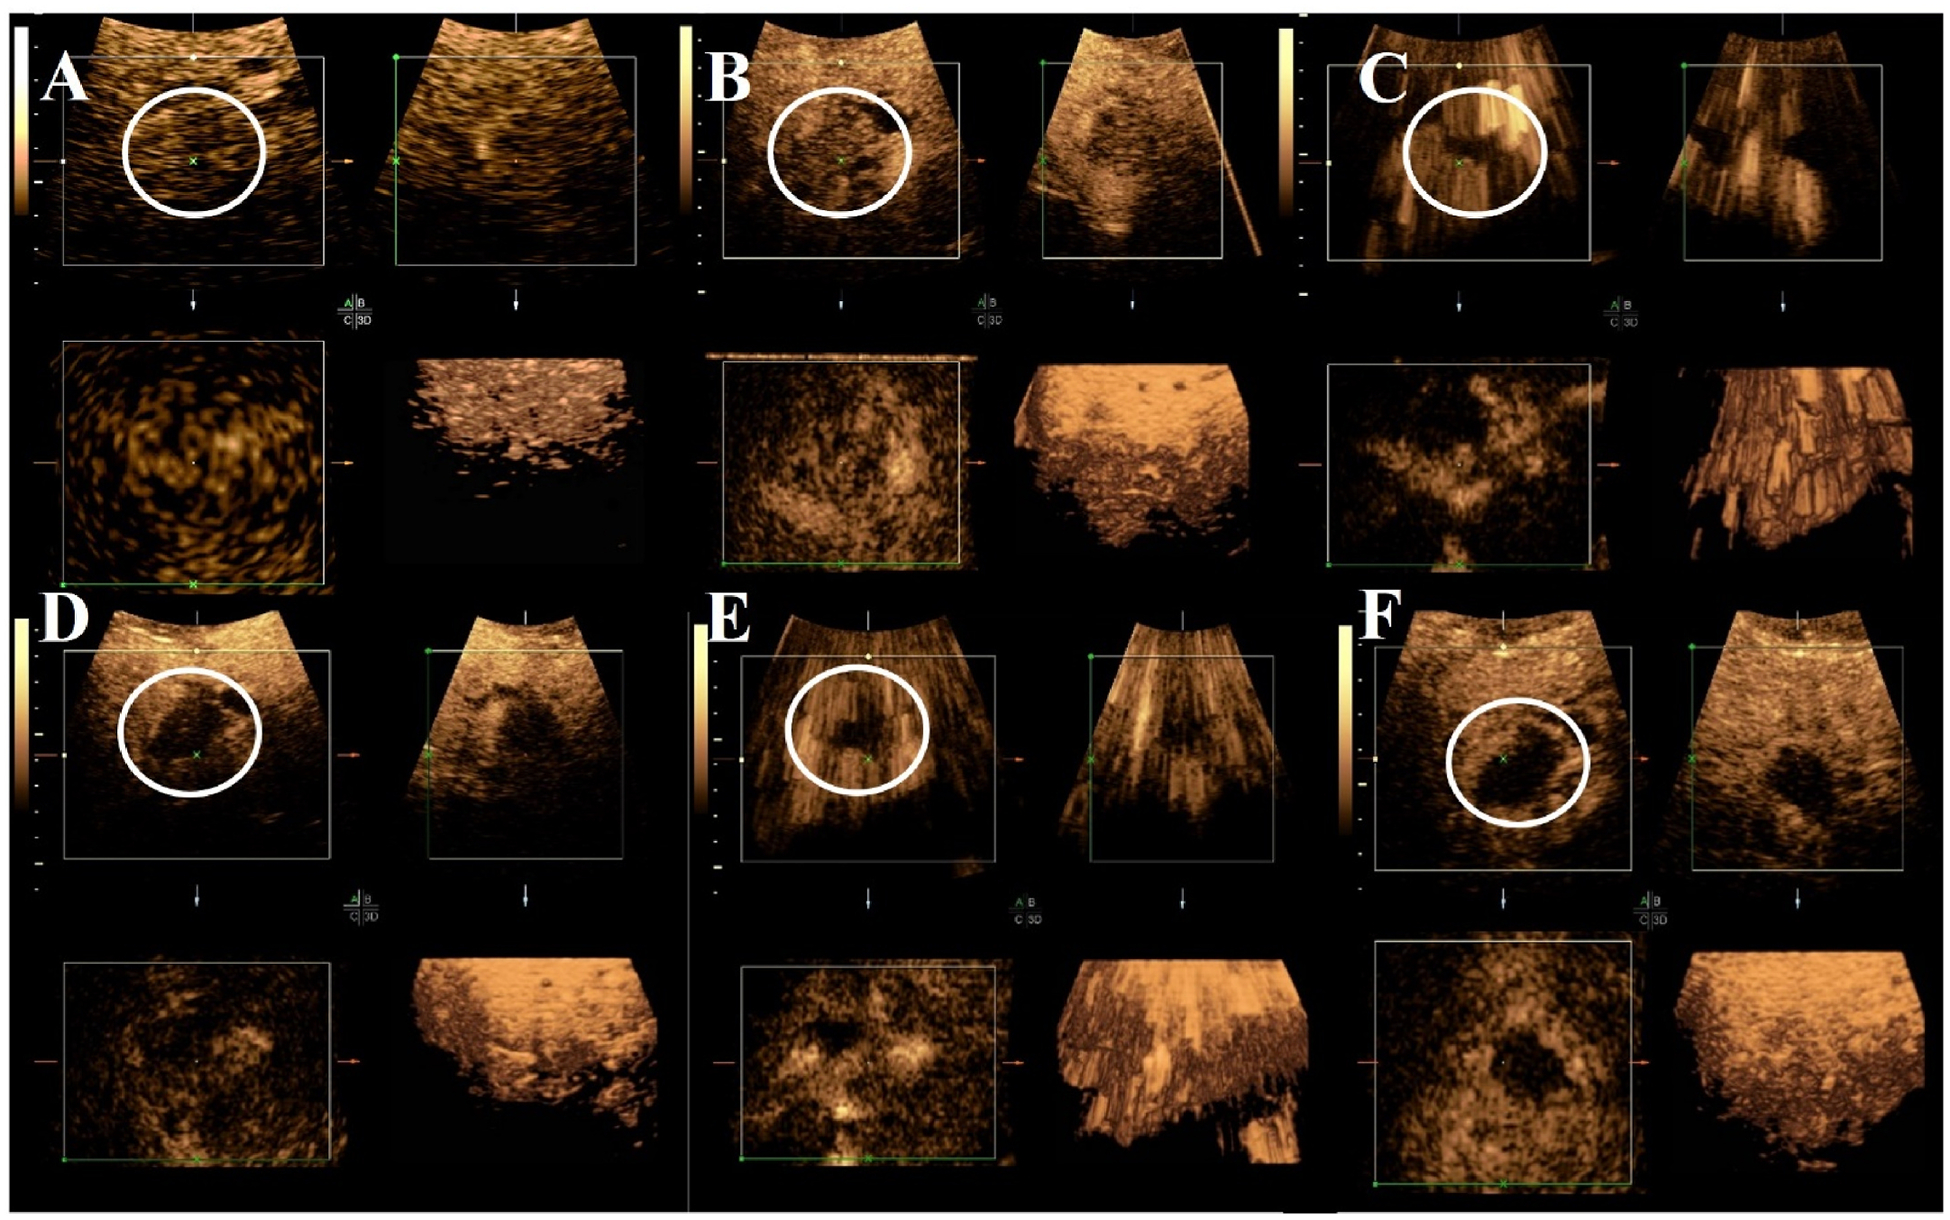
An example of serial 3D UTMD imaging. 3D UTMD CEUS imaging of an intrahepatic cholangiocarcinoma study participant with a 4.1 cm segment 2/3 tumor. Successive 3D CEUS imaging over four timepoints demonstrates (A) complete enhancement two weeks prior to Y90-TARE. (B) CEUS imaging immediately post Y90-TARE with internal enhancement. (C) UTMD high intensity pulse (ISPTA = 176 mW/cm^2^) immediately post Y90-TARE. (D) 1 week post Y90-TARE with minimal contrast enhancement noted within the intrahepatic cholangiocarcinoma. (E) UTMD high intensity pulse (ISPTA = 152.7 mW/cm^2^) at 1 week post Y90-TARE. (F) 2 weeks post Y90-TARE no contrast enhancement is noted within the intrahepatic cholangiocarcinoma, correlating with complete response observed on mRECIST. White circle corresponds to intrahepatic cholangiocarcinoma tumor. UTMD = ultrasound-triggered microbubble destruction, CEUS = contrast-enhanced ultrasound, Y90-TARE = yttrium-90 transarterial radioembolization, ISPTA = intensity spatial peak temporal average, mRECIST = modified response evaluation criteria in solid tumors.
